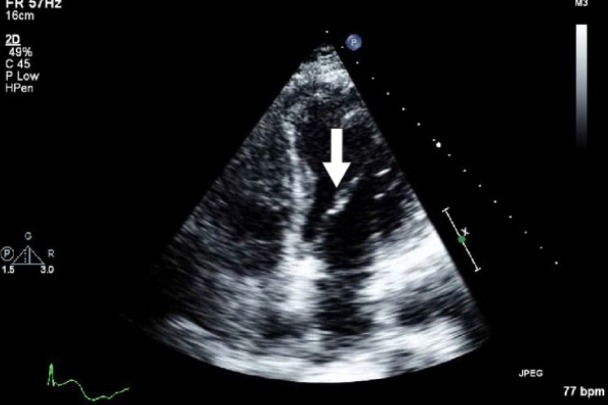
Echocardiogram showing mild mitral regurgitation and a moderate sized vegetation on the anterior mitral valve leaflet.
